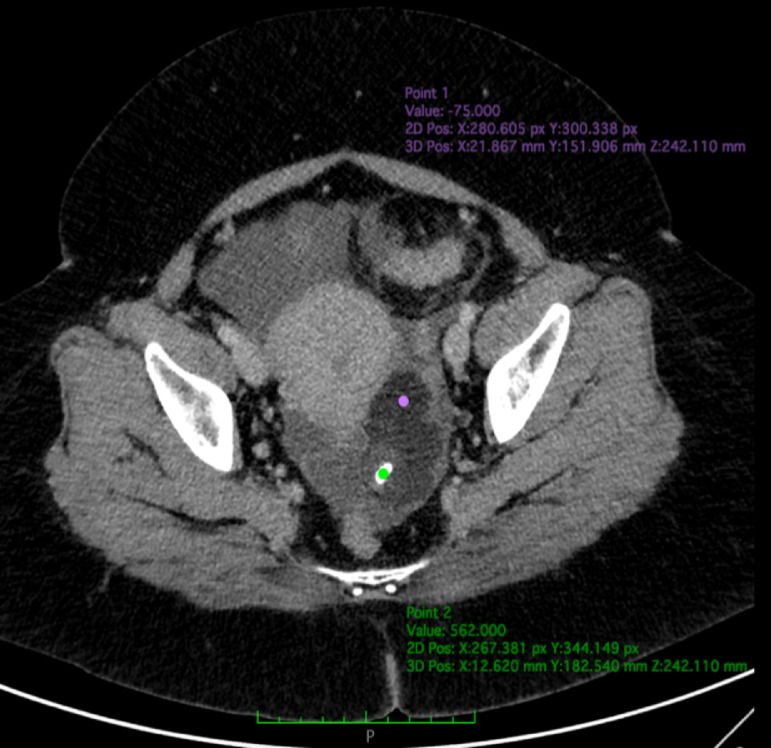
Axial CT view in venous phase, showing a mass with a fat-dominant (-75 HU, purple dot) component, minimal solid component, and calcification (562 HU, green dot), measuring 5.4 × 3.6 × 7.0 cm on the left ovary. Ascites is apparent in the abdominal and pelvic cavities (asterisk) (Color version of the figure is available online.)
